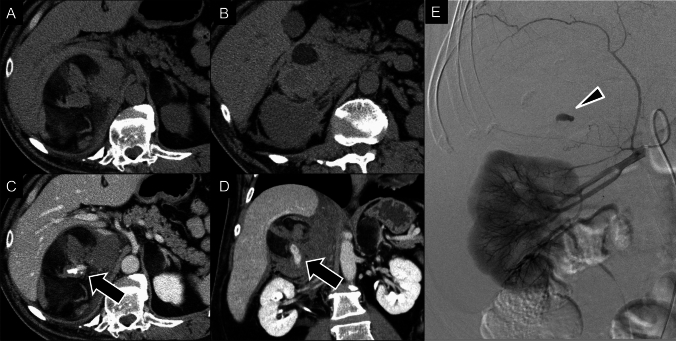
Retroperitoneal hemorrhage from adrenal myelolipoma. A 38-year-old male presented with right-upper quadrant pain. A, B Unenhanced computed tomography (CT) images showing a right-adrenal mass containing macroscopic fat, suspected to be an adrenal myelolipoma or cortical adenoma with myelolipomatous degeneration. The tumor had a growth rate of 8 mm/year prior to this imaging. C, D Enhanced CT showing contrast media extravasation within the mass, confirming active bleeding (arrows). E Right-renal angiography showing active extravasation from the right-inferior adrenal artery (arrowhead), which was successfully treated with glue embolization
